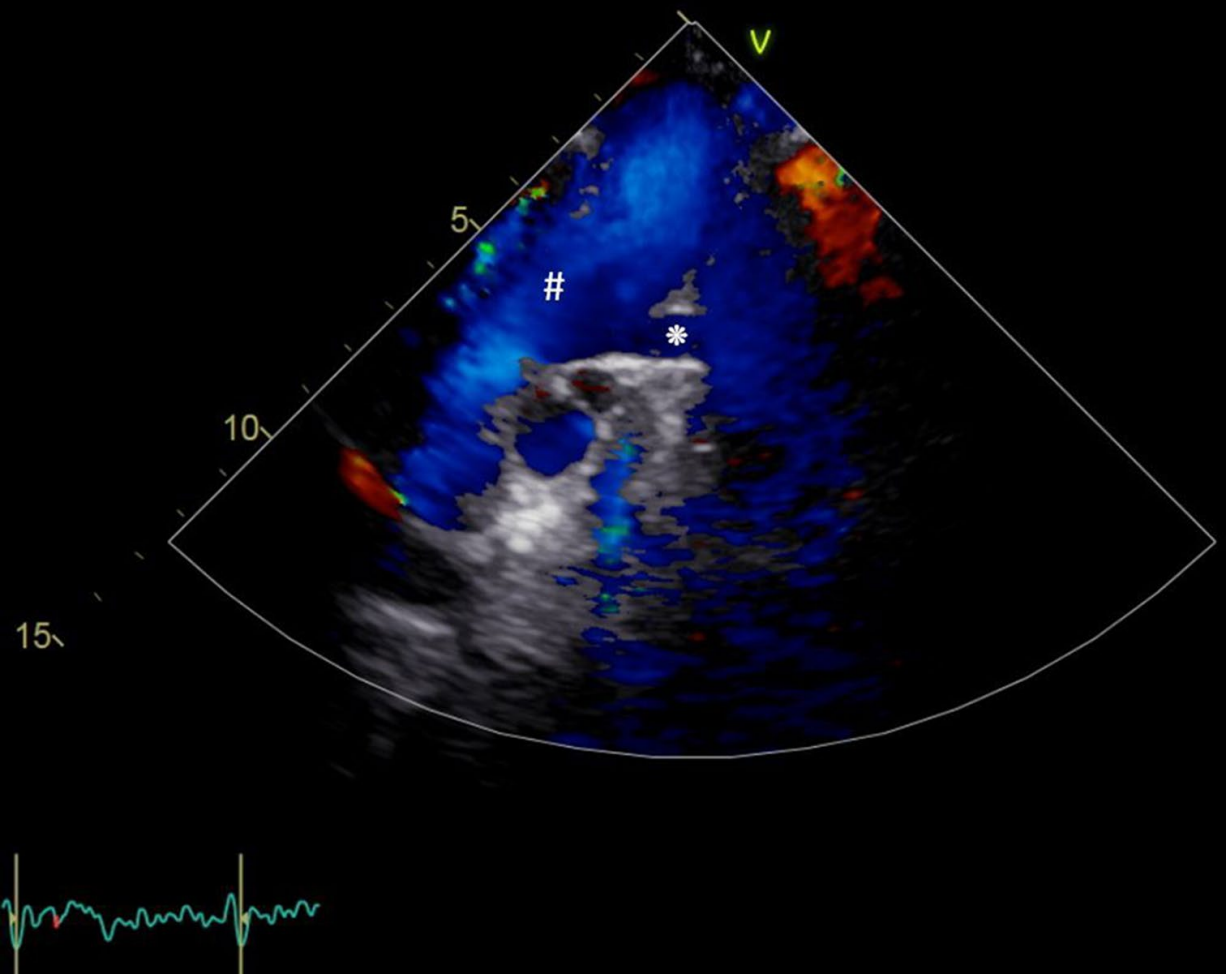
Patient B’s postoperative transthoracic echocardiography and vascular ultrasound exam showcasing a suspected anomalous pulmonary vein connection (APVC) (*) conducted by the department of pediatric cardiology and congenital heart diseases; #: innominate vein
